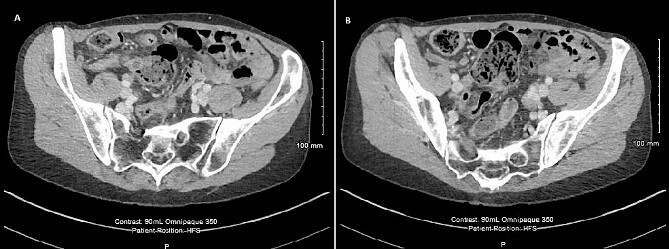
CT scan of the abdomen showing (A) 10 mm low attenuation lesion at the base of appendix and (B) mildly thick walled appendix containing a small volume of fluid.
